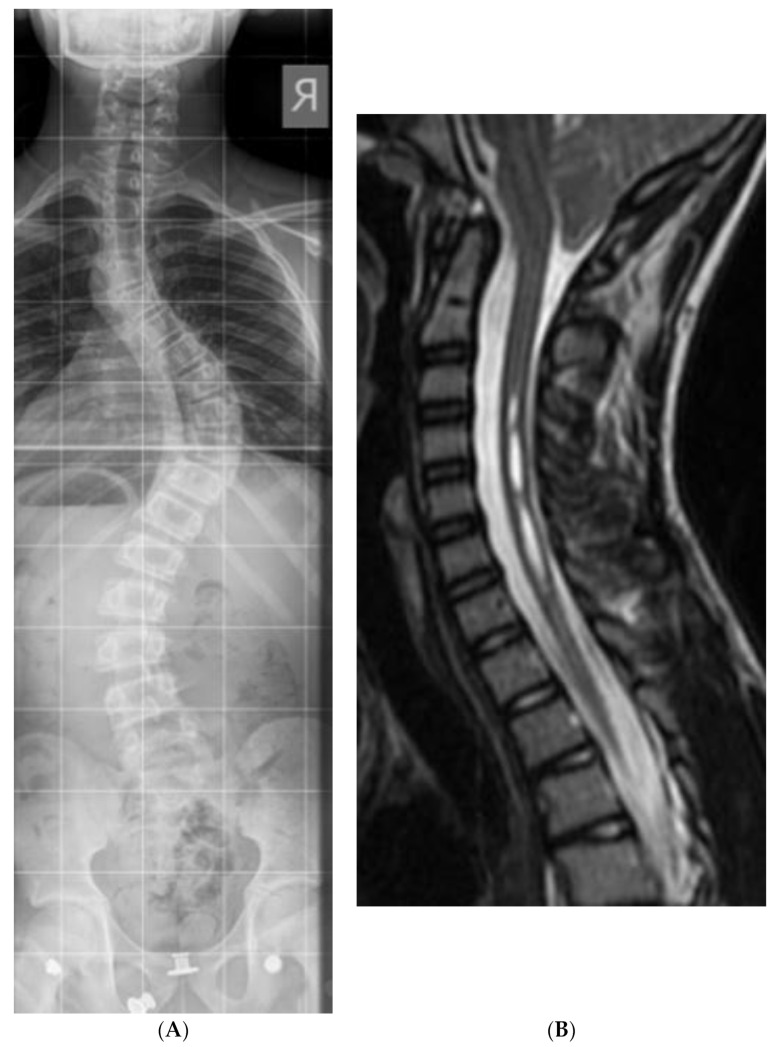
(A) Thirteen years old AIS patient with CM-1—AP Spine. (B) Thirteen years old AIS patient with CM-1—Cspine MRI.
